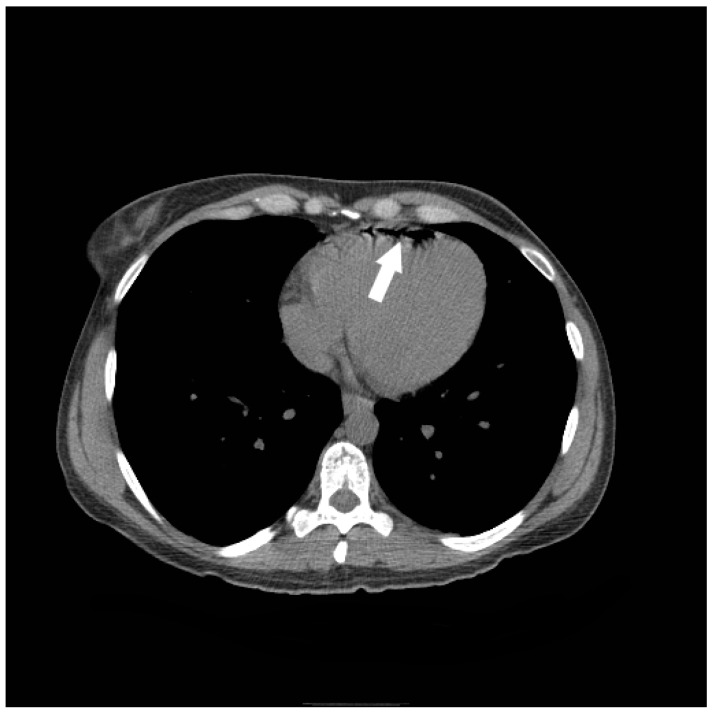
A 35-year-old woman had an incidental finding of air in the right ventricle on non-contrast CT abdomen performed for renal stone evaluation. The patient was asymptomatic and an IV line had been placed approximately 1 hour earlier.
